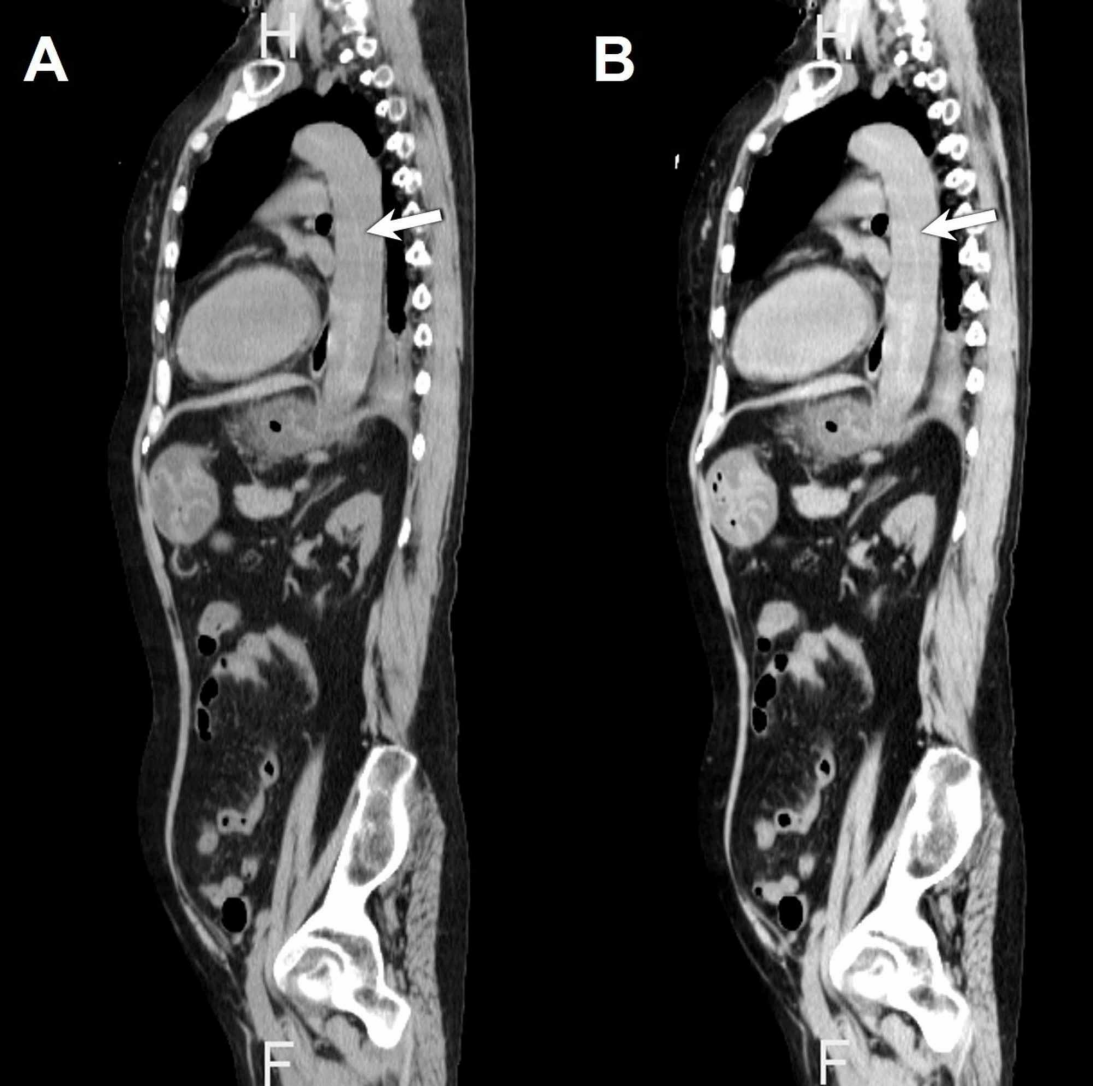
Precontrast CT scans of the aorta (A) The descending aorta was dissected (arrow). (B) The aortic dissection did not worsen during the hospitalization (arrow).
